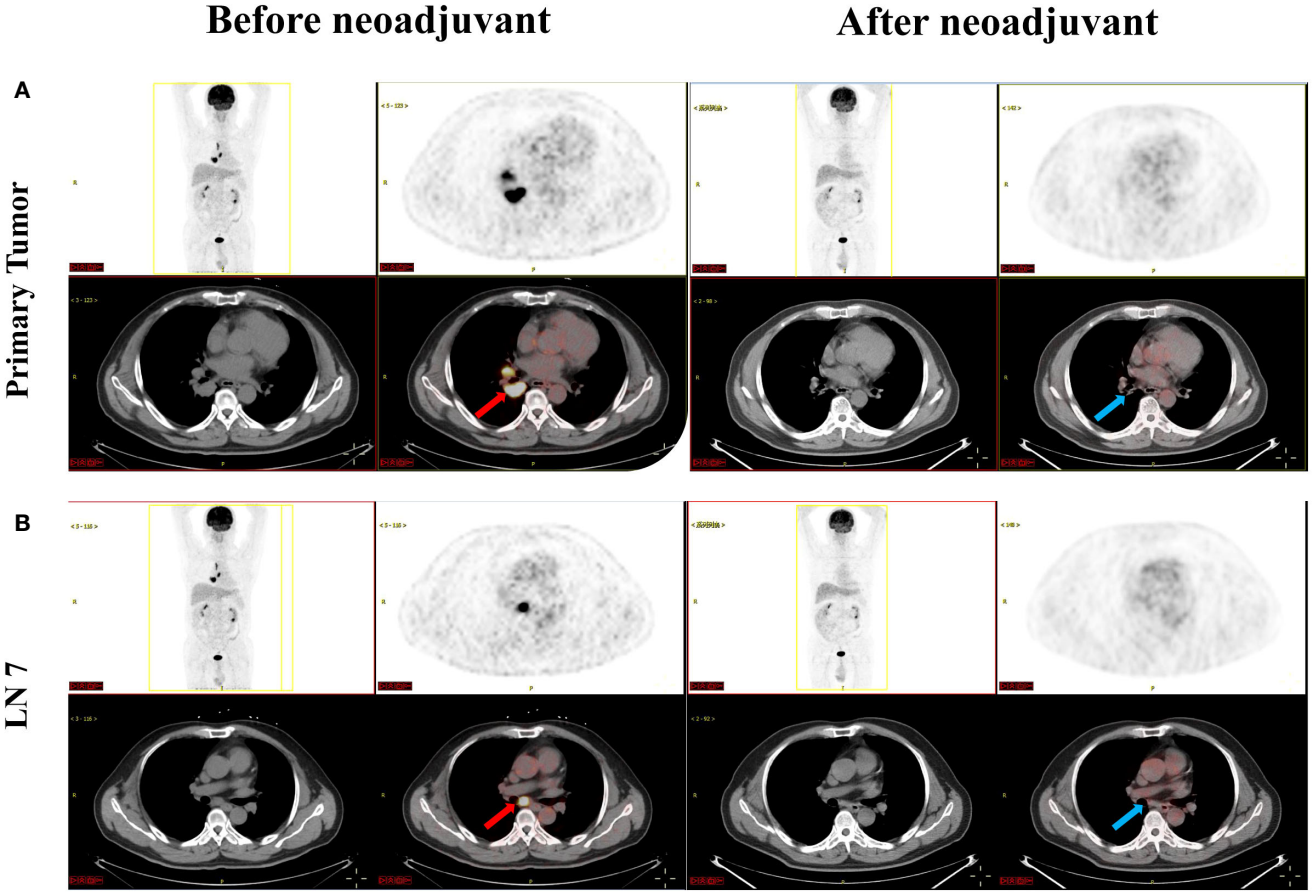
Images before (red arrow) and after (blue arrow) neoadjuvant camrelizumab plus chemotherapy treatment. (A) showed PET-CT of the primary tumor; (B) showed PET-CT of the 7th station lymph nodes. LN 7, 7th station lymph nodes; PET-CT, positron emission tomography-computed tomography.
